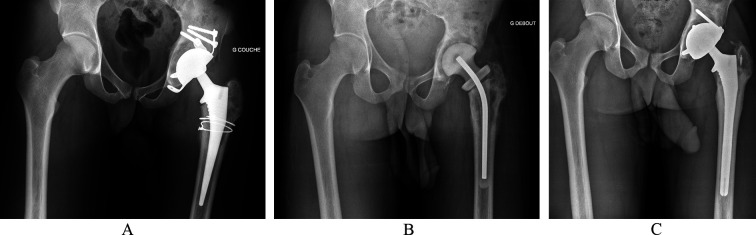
First case of Hip chronic Prosthetic Joint Infection. Male, 18 years-old, history of THA for avascular necrosis (Sickle-cell disease) 6 months ago. Pathogen: Methicillin-sensitive *Staphylococcus aureus*. (A) Pre-operative AP X-ray. (B) Preformed articulated spacer. (C) 4-year follow-up AP X-ray.
